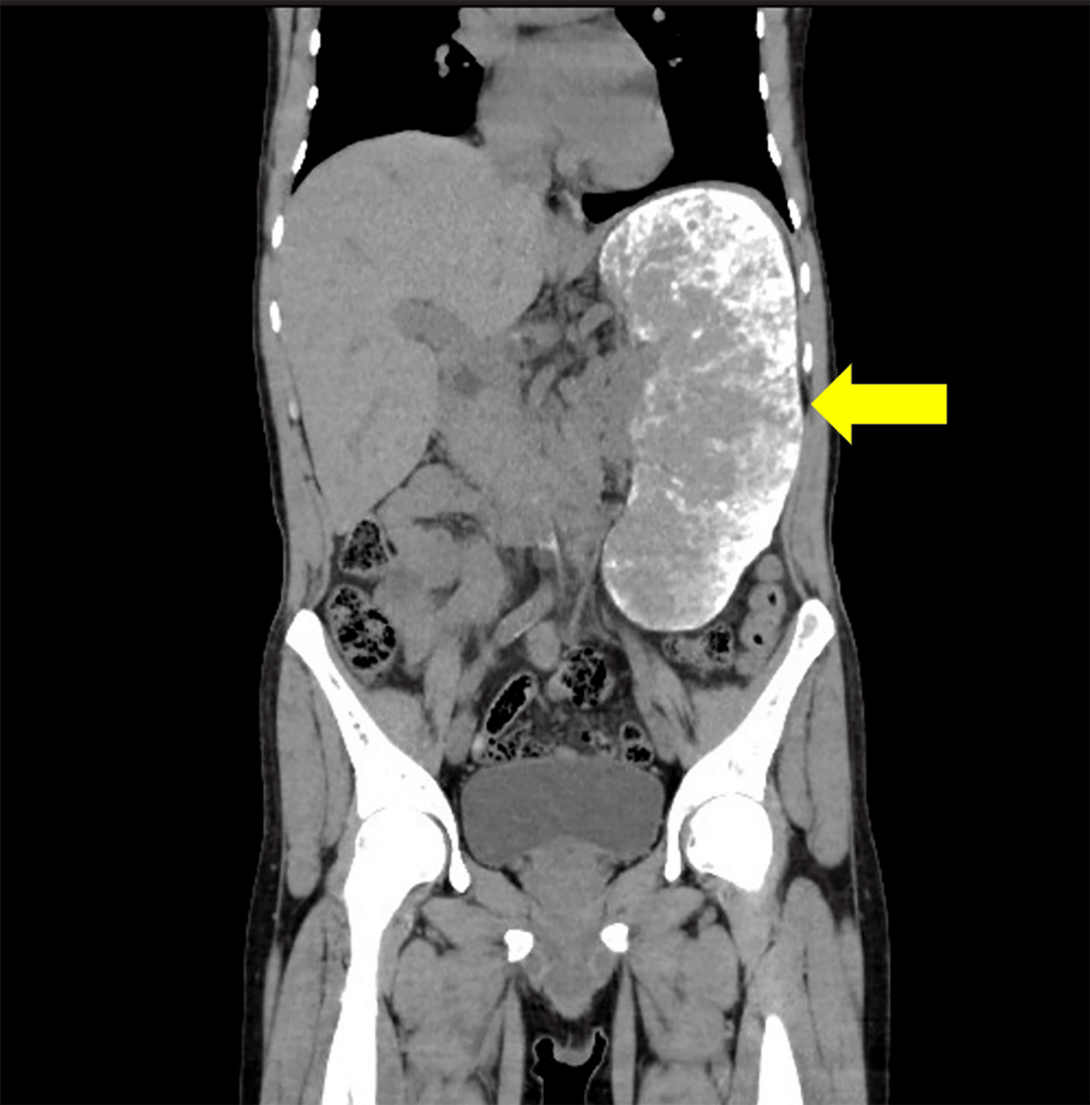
Computed tomography revealed marked splenomegaly, with diffuse high-density areas containing hemosiderin and calcification deposits.
